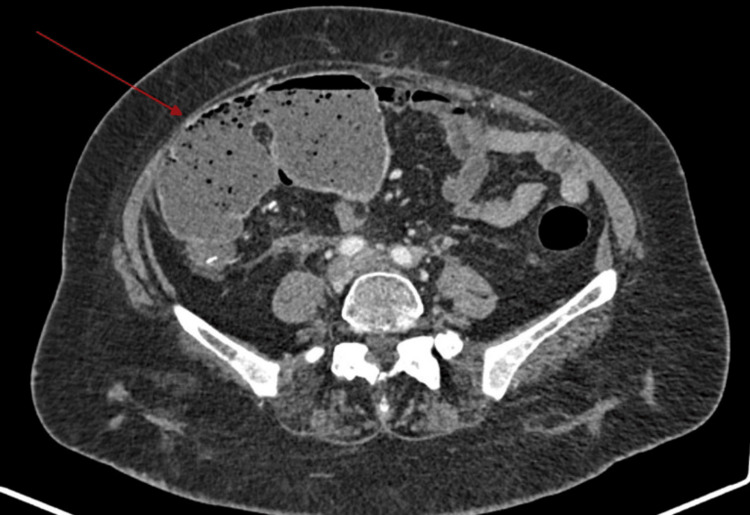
Abdomino-pelvic CT (axial view) demonstrating cecal volvulus (red arrows)
